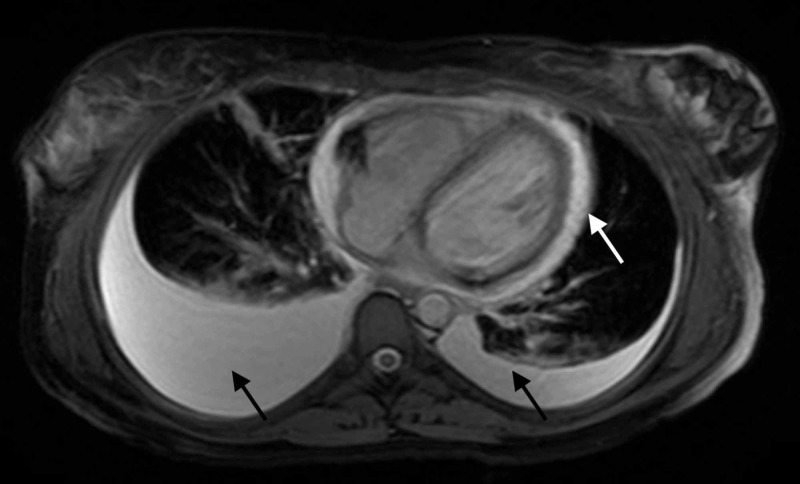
MRI of the abdomen without contrast Black arrows showing bilateral pleural effusions, moderate on the right and small on the left. White arrow showing moderate pericardial effusion.
